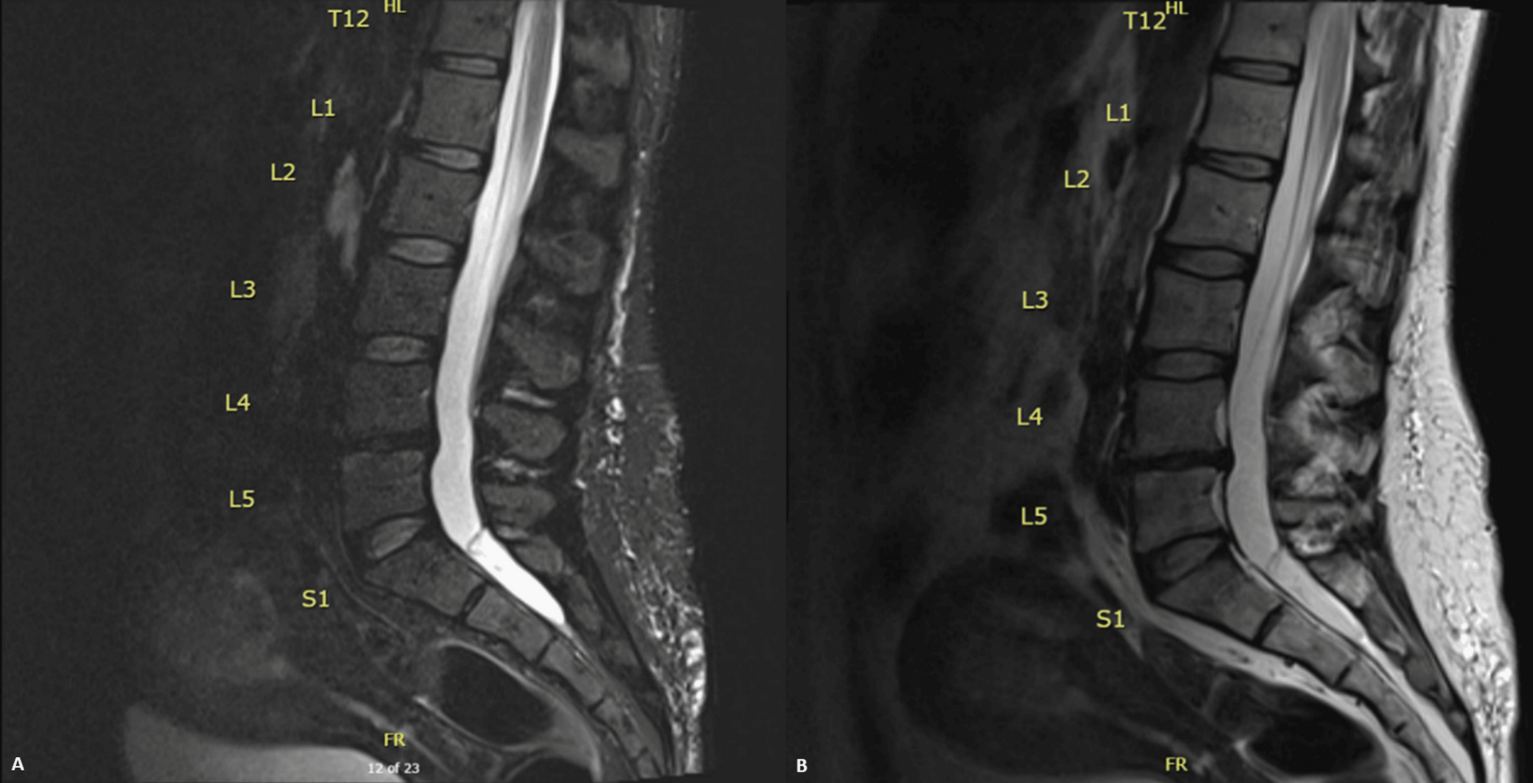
Initial MRI lumbar spine sagittal view. From left to right, image A shows initial findings of the patient from a lumbar MRI short tau inversion recovery sequence (STIR) of degenerative disc changes at L4-L5 and peripherally located nerve roots, and image B shows the T2 sequence.
